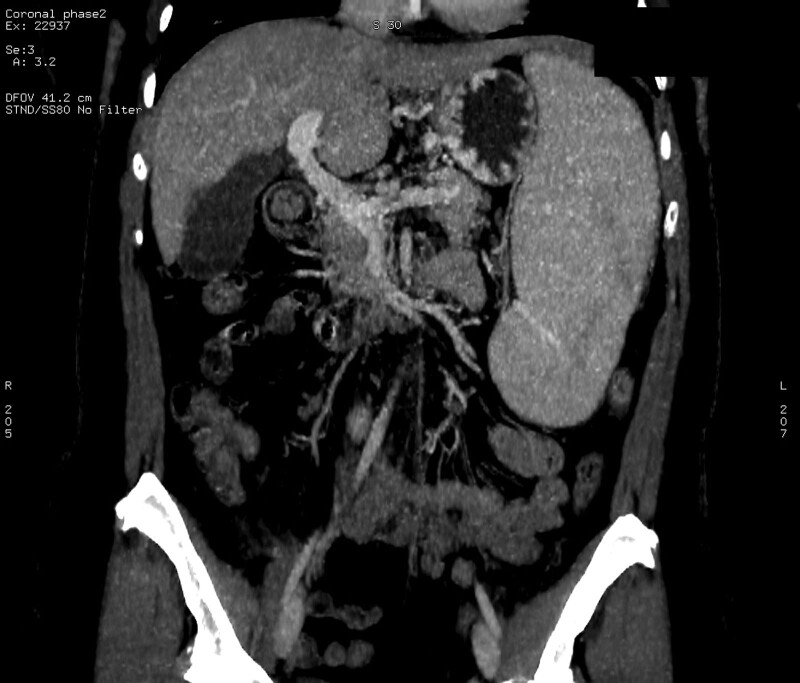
Near-complete recanalization of the superior mesenteric vein on follow-up contrast-enhanced CT scan 2 months later. CT = computed tomography.
